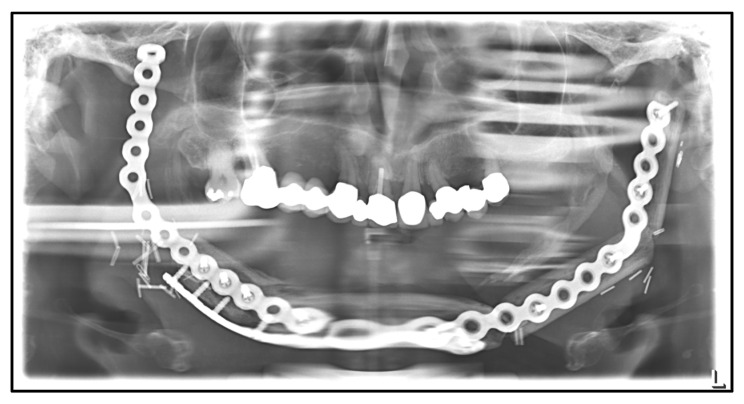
Dental X-ray after total mandibular resection and reconstruction with a fibular free flap. Fractured hand-bent plate in the right anterior region and supporting reconstruction plate on the basal rim of the neo-mandible.
